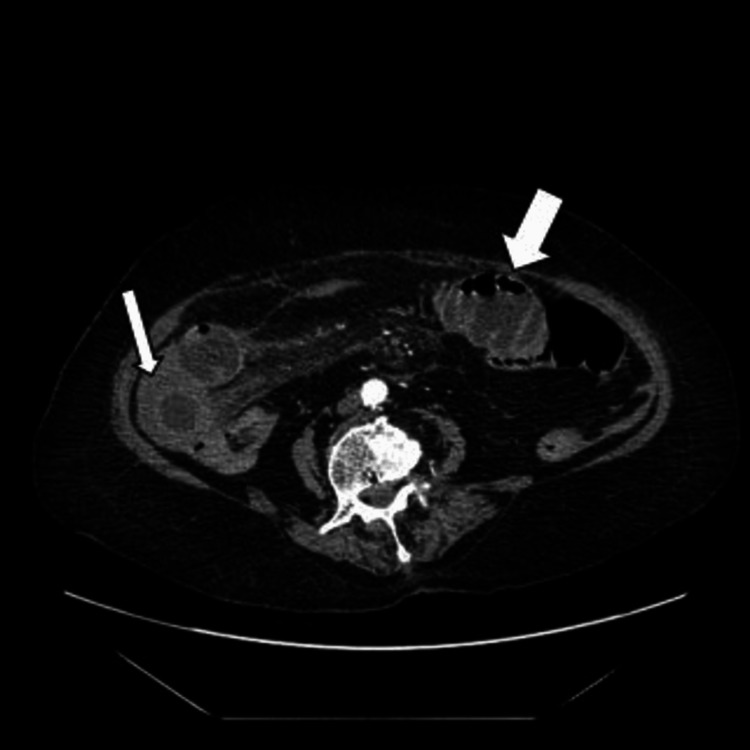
A contrast CT shows a parietal hematoma (narrow arrow) responsible for a gallbladder occlusion (wide arrow) with the infiltration of the mesenteric fat in front.
